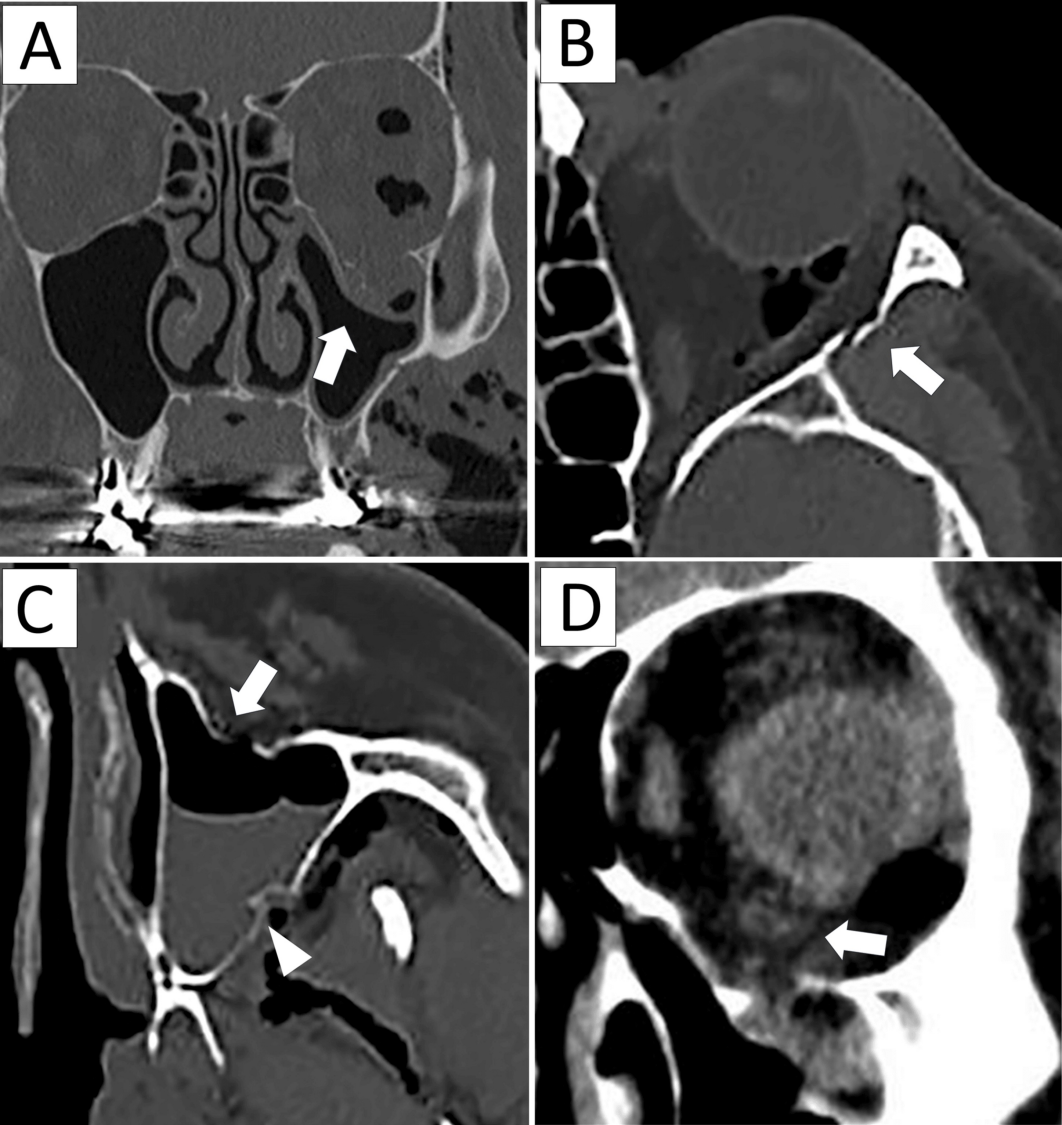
CT images A. Herniation of orbital contents into the maxillary sinus (arrow). B. Fracture of the lateral orbital wall (arrow). C. Fractures of the anterior wall (arrow) and lateral wall (arrowhead) of the maxillary sinus, with hematoma in the sinus. D. The inferior rectus muscle (arrow) remained within the orbit and did not herniate into the maxillary sinus.
